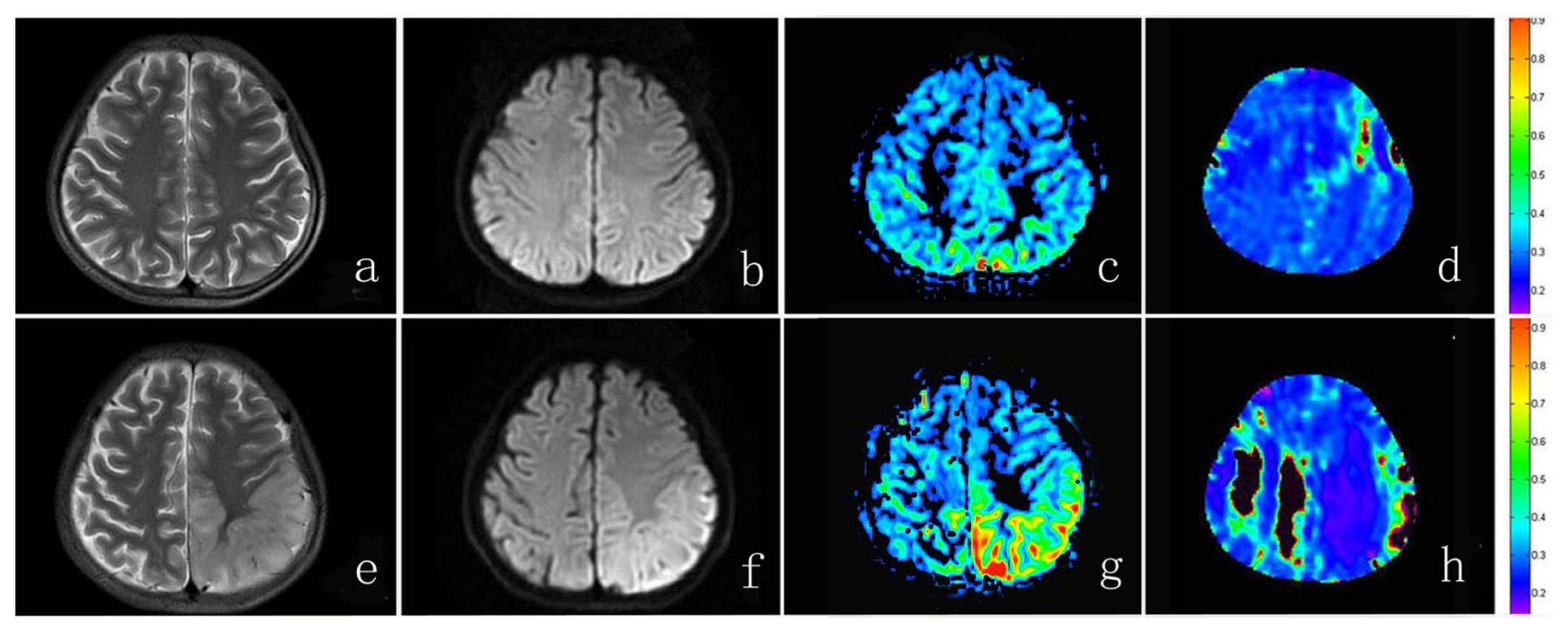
MRI in a 14-year-old boy with MELAS. The top row shows images at the interictal phase. T2WI image (A) , DWI image (B) and CBF map (C) are unremarkable. OEF map (D) shows global decreased OEF value relative to controls. The bottom row shows images at the acute phase, 9 days after the stroke-like episode. High signal and swelling of left parietal lobe can be seen on T2WI image (E) and DWI image (F). Increased CBF is depicted in the lesion on the CBF map (G). The OEF is markedly decreased across the brain, especially in the lesion region (H). The regions with low SNR are marked as black to be excluded from measurement.
